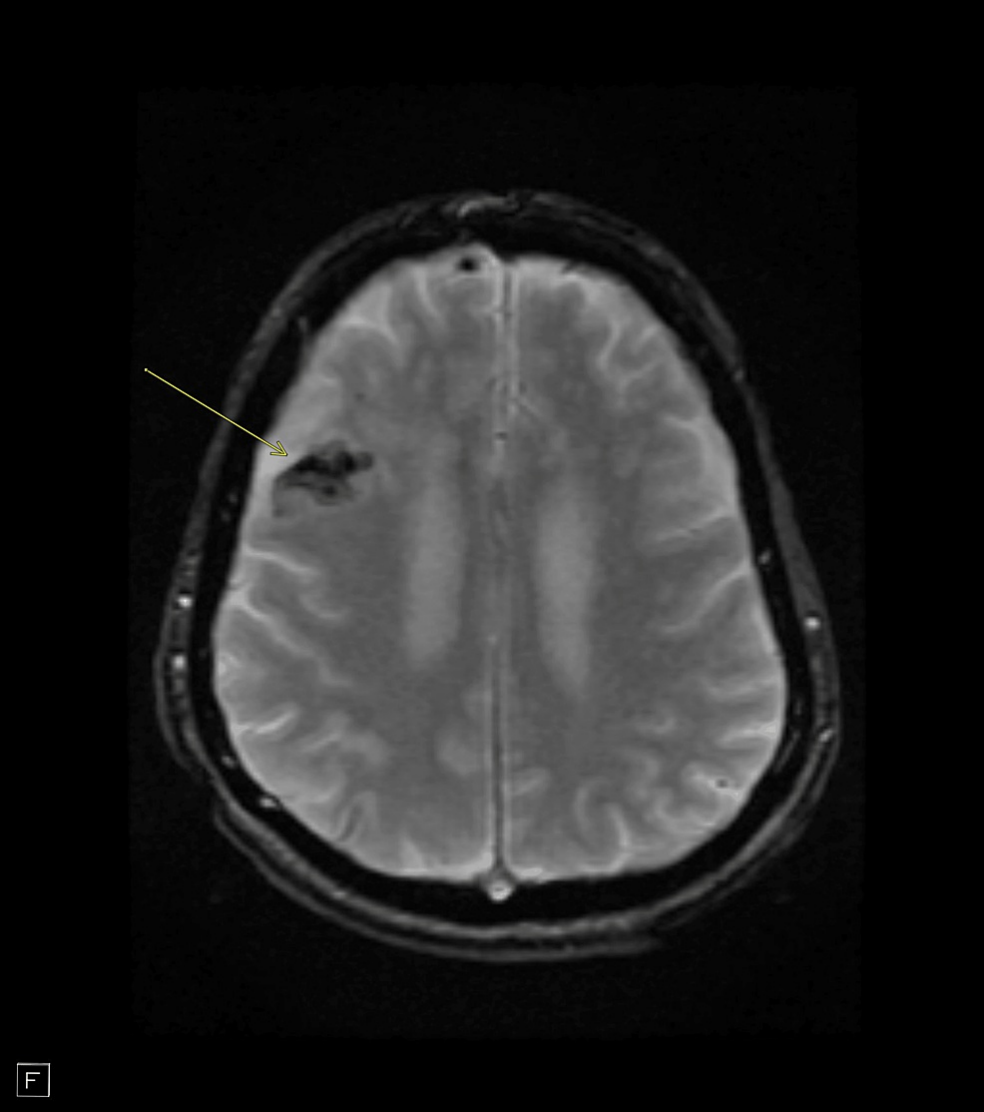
MRI brain without IV contrast showing a new area of right frontal subarachnoid hemorrhage (axial T2 image, arrow). Multiple additional foci of restricted diffusion reflecting embolic infarcts were noted in other cuts.
